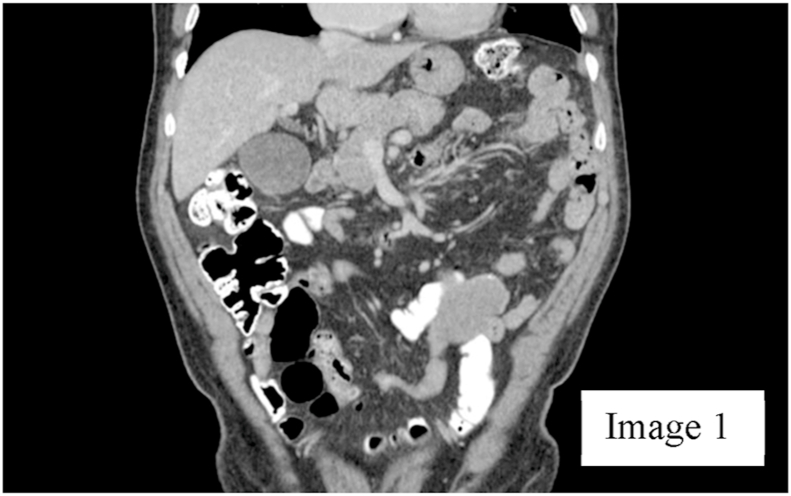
Coronal plane of CT scan of abdomen.
